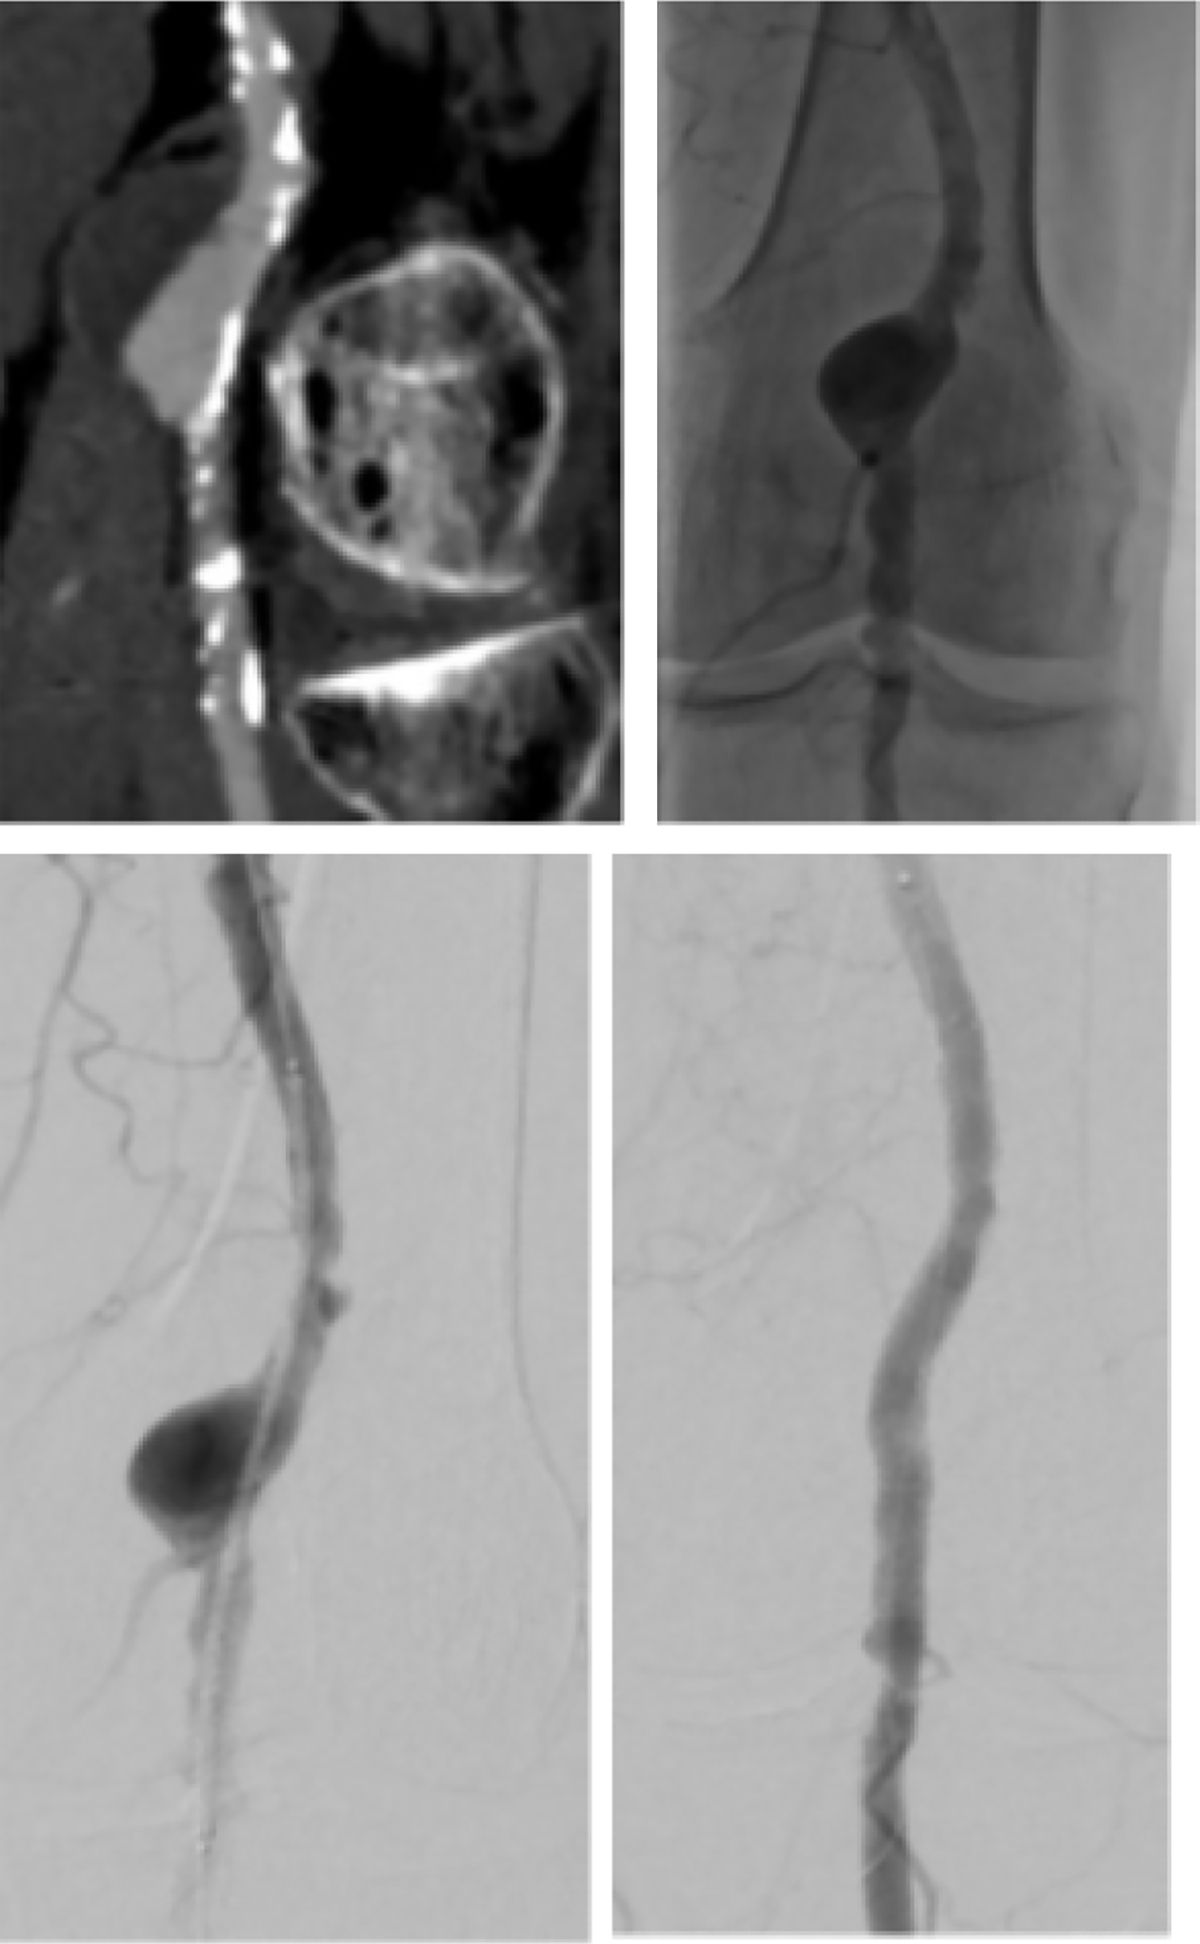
A: CTA of the left popliteal artery. The popliteal artery shows an aneurysm with partial thrombosis, B: Angiogram shows the perfused PAA, C: Positioning of the endoprosthesis, D: perfused Endoprosthesis, some retrograde perfusion is seen, this is accepted as perfusion of the aneurysm is sealed by the endoprosthesis. PAA: Popliteal artery aneurysm
